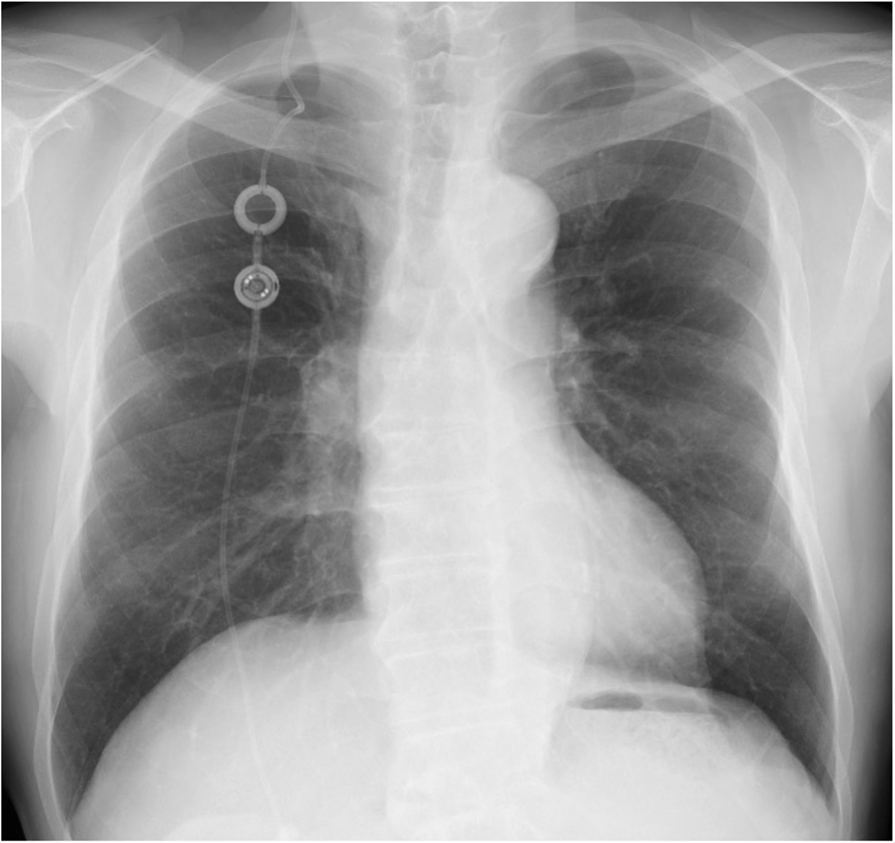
Preoperative chest X-ray. Ventriculoperitoneal shunt reservoir and valve located anteriorly in the thoracic region
